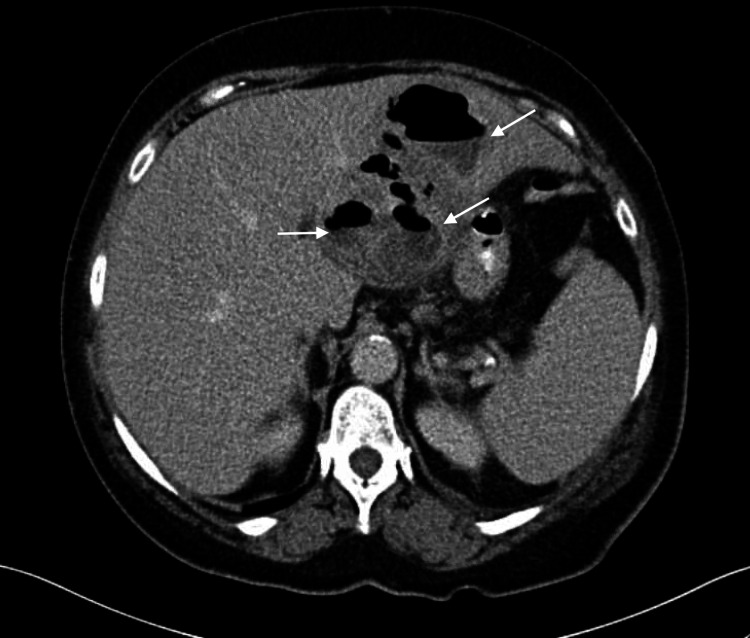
CT scan of the abdomen with intravenous contrast shows liver abscess of the left lobe with multiple fluid levels on the sagittal plane (white arrow)
